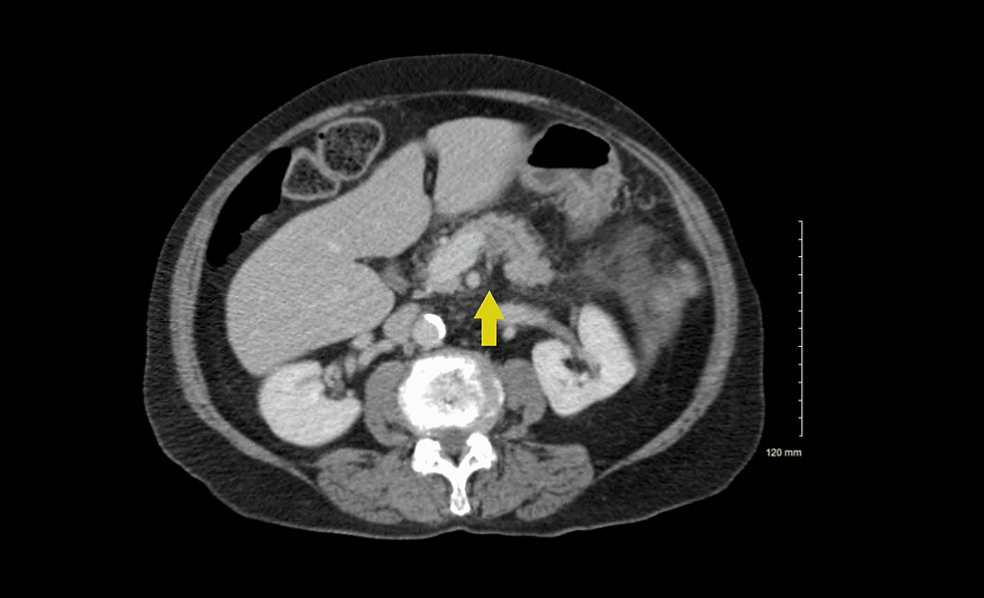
CT of abdomen pelvis with contrast Arrow is demonstrating pancreatitis.
